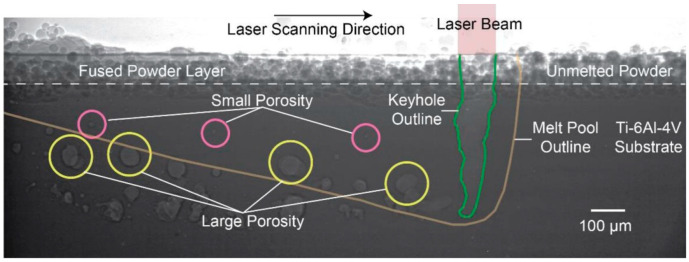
In situ X-ray imaging showing defect formation and melt pool outline detection in LPBF. Figure is reprinted from [237] under Creative Commons Attribution License (CC BY).
